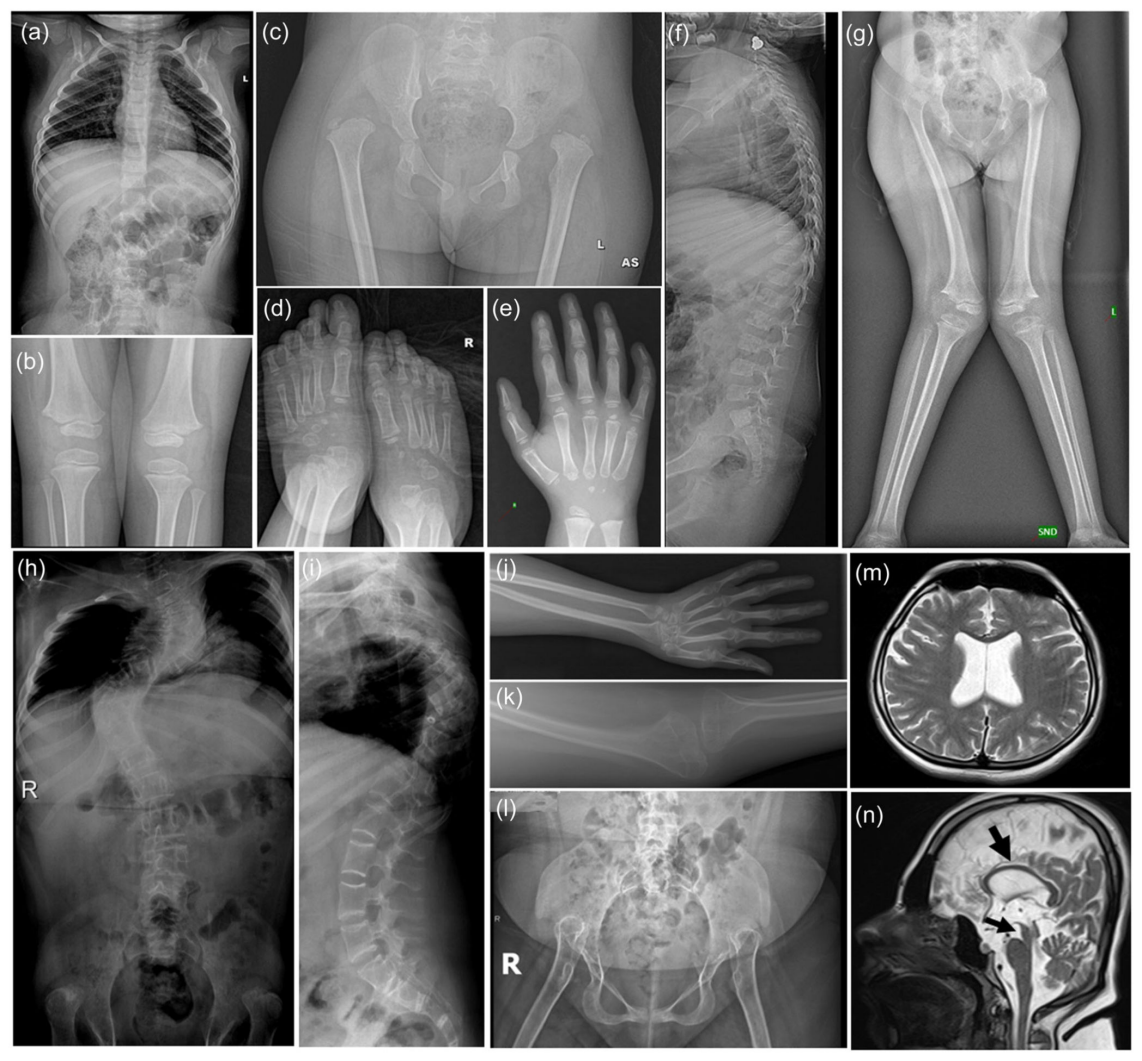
Radiographs of Patient 1 (P1) at age 3 years show narrow interpedicular distance in lumbar vertebrae, slender ribs (a), small epiphyses and irregular metaphyses at the knee (b). Bilateral hip dislocation, small femoral epiphyses and narrow acetabulum are evident (c). Slender metatarsals/leptodactyly in hands and feet and delayed carpal bone ossification in hands are noted (d and e). She has exaggerated lumbar lordosis and irregular vertebral end plates (f). Bilateral dislocation of knees and genu valgum is observed in proband at the age of 5 years and 7 months (g). Radiographs of Patient 2 (P2) at 13 years of age show severe thoracic kyphoscoliosis, slender ribs, increased lumbar vertebra body heights, and irregular and sclerosing endplates (h and i). Radiographs of limbs (j) show diffuse osteopenia and slender long and short tubular bones. Note that the metacarpals are leptodactylic and knee (k) and hip joints (l) are dislocated. Bilateral shallow acetabular fossa, short iliac wings, metaphyseal widening and epiphyseal irregularity, enlarged distal phalangeal tufts, and distal radioulnar subluxation are also observed (l). Brain magnetic resonance imaging (m and n) of P2 at 13 years of age (axial and sagittal T2-weighted brain magnetic resonance images) revealed hydrocephalus, brachycephaly, thin corpus callosum (thick arrow), and hypoplastic mesencephalon (thin arrow).
